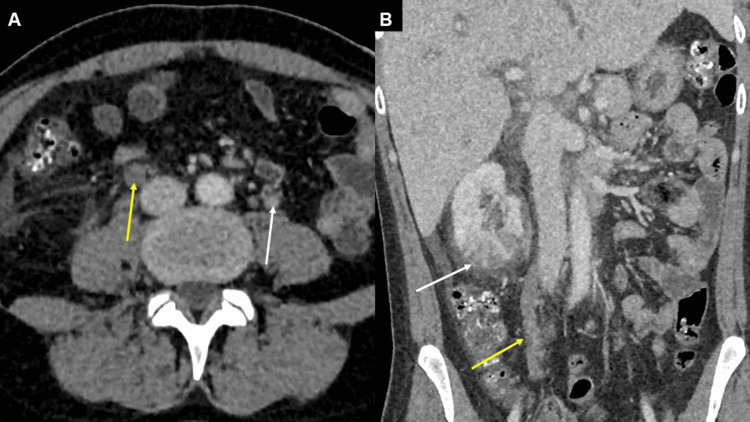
(A) Axial computed tomography of the abdomen with intravenous contrast in portal venous phase demonstrating asymmetrically prominent right ovarian vein with absent luminal contrast opacification (yellow arrow) and mild surrounding fat stranding. Note the normal opacification of the left ovarian vein (white arrow). (B) Coronal computed tomography again shows the absent contrast opacification of the right ovarian vein (yellow arrow), consistent with ovarian vein thrombosis in the appropriate clinical setting. Also note the striated right renal cortical enhancement with perinephric fat stranding (white arrow), representing acute pyelonephritis.
